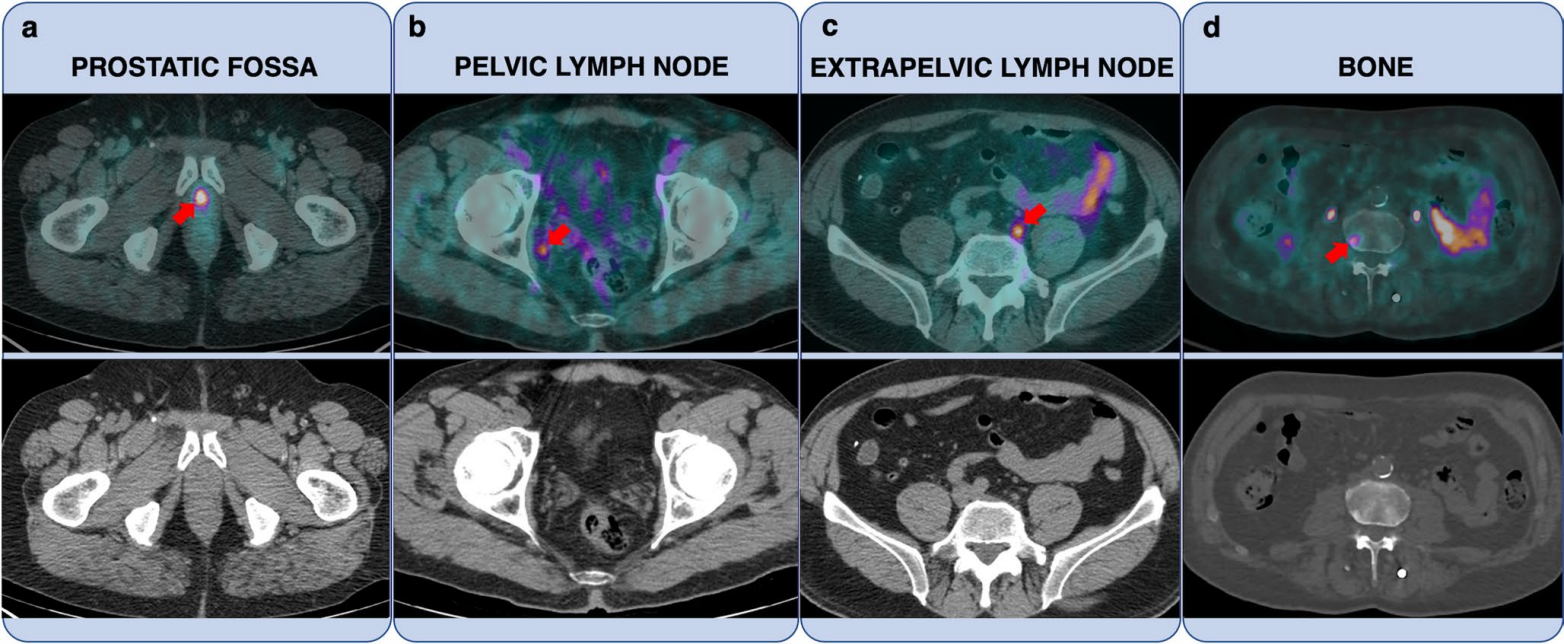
Examples of PSMA-PET/CT positive disease. (a) A 63-year-old patient with biochemical recurrence (BCR) (PSA = 0.37 ng/mL) of PCa occurred 9 months after radical prostatectomy (RP). PSMA PET identified a focal uptake in the prostatic fossa (red arrow). (b) A 73-year-old patient with BCR (PSA = 0.23 ng/mL) 24 months after RP. PSMA PET identified a pelvic lymph node in the right obturator region (red arrow). (c) A 67-year-old patient with BCR (PSA = 0.91 ng/mL) 8 months following RP. PSMA PET revealed metastatic disease in the left common iliac region (red arrow). (d) A 71-year-old patient with BCR (PSA = 0.31 ng/mL) 26 months after RP. PSMA PET showed bone disease in the right hemibody of D5 (red arrow)
